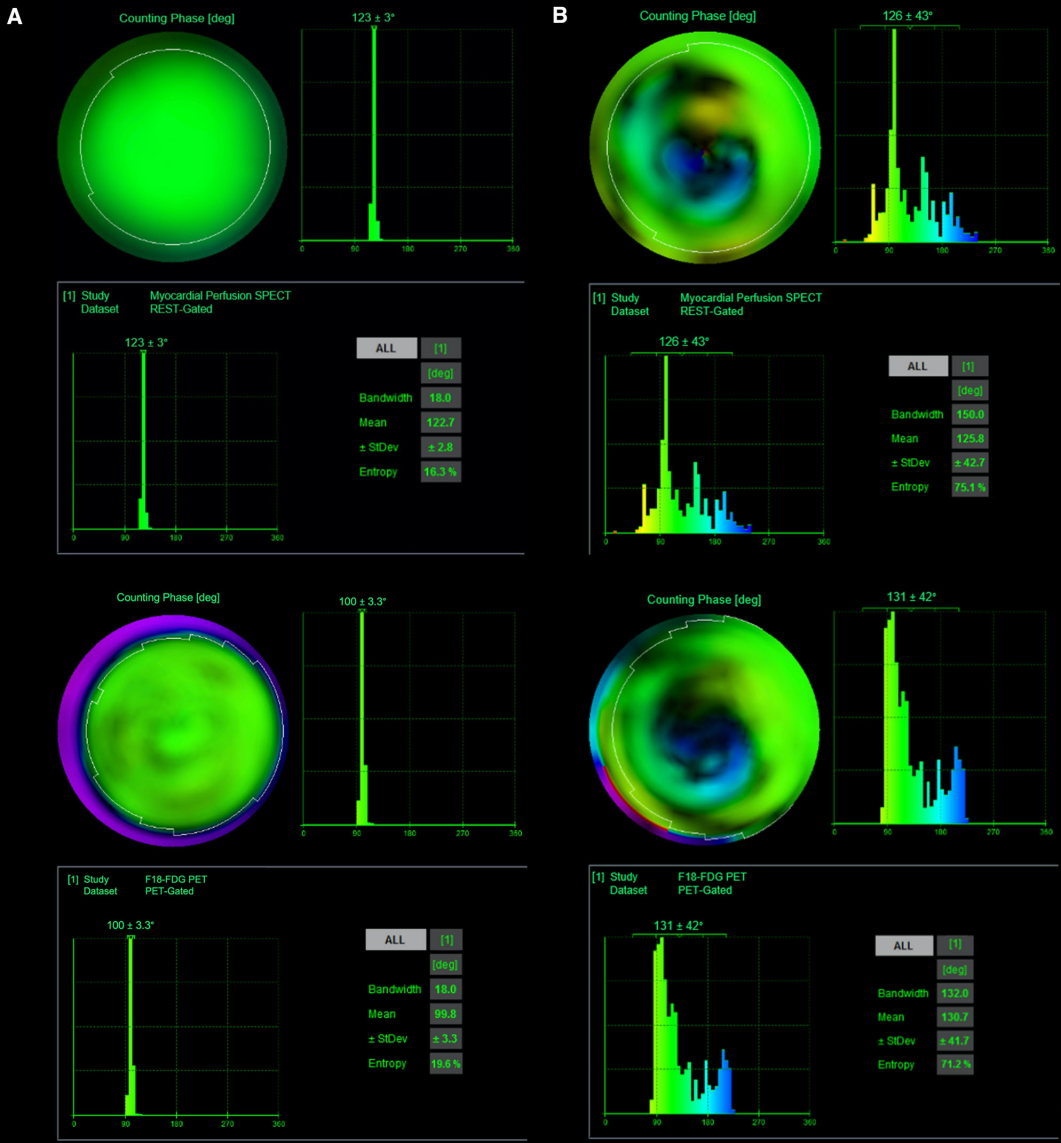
Phase analysis with QGS. A shows the SPECT (upper image) and PET (lower image) phase analysis of the same patient without dyssynchrony. B shows the SPECT (upper image) and PET (lower image) phase analysis of the same patient with dyssynchrony
